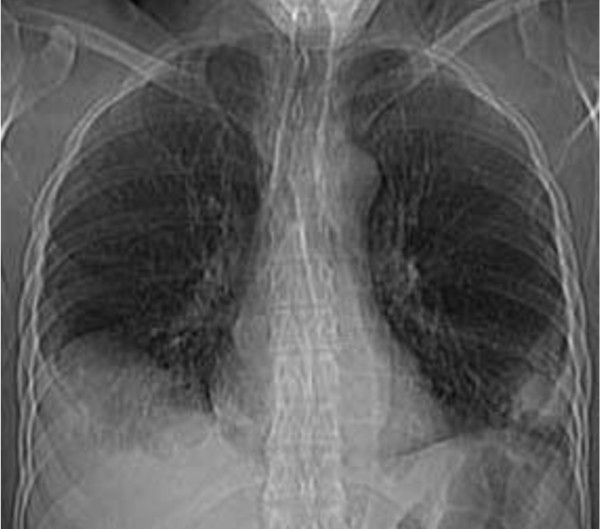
Chest computed tomography (CT) examination of the patient. This chest CT showed pleural effusion on the right pulmonary base.
